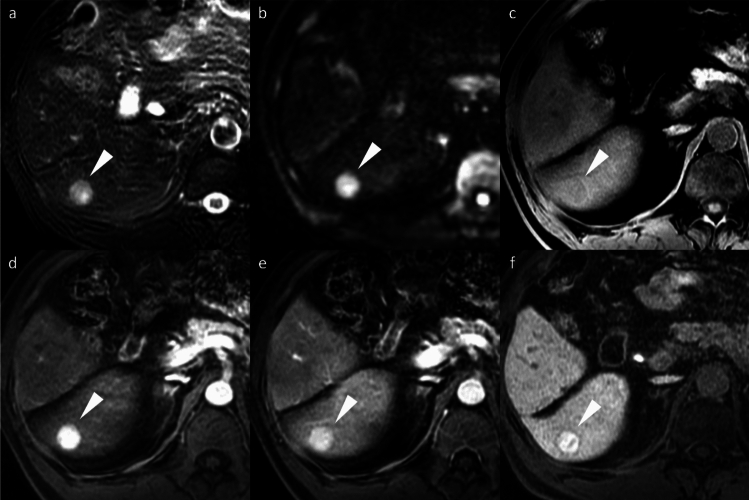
Hyperintense hepatocellular carcinoma in the hepatobiliary phase in a 58-year-old man. A nodule (arrowheads) located in the posterior segment appears clearly hyperintense on fat-suppressed T2-weighted images (a) and demonstrates diffusion restriction on diffusion-weighted images (b). The nodule displays slight hypointensity on the pre-contrast image (c), non-rim arterial phase hyperenhancement (d) without portal venous washout (e), and clear hyperintensity in the hepatobiliary phase (f). The nodule was pathologically proven to be hepatocellular carcinoma following surgical resection
